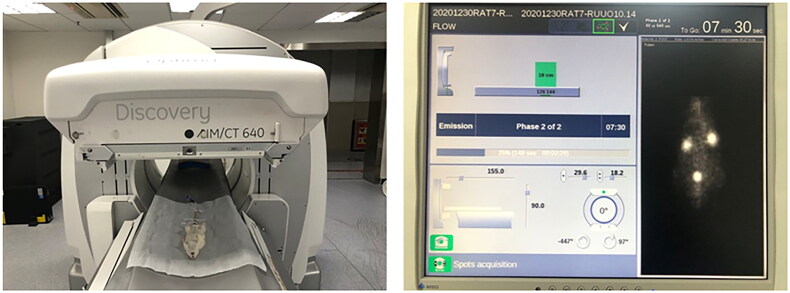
SPECT-procedure for renal function assessment.
